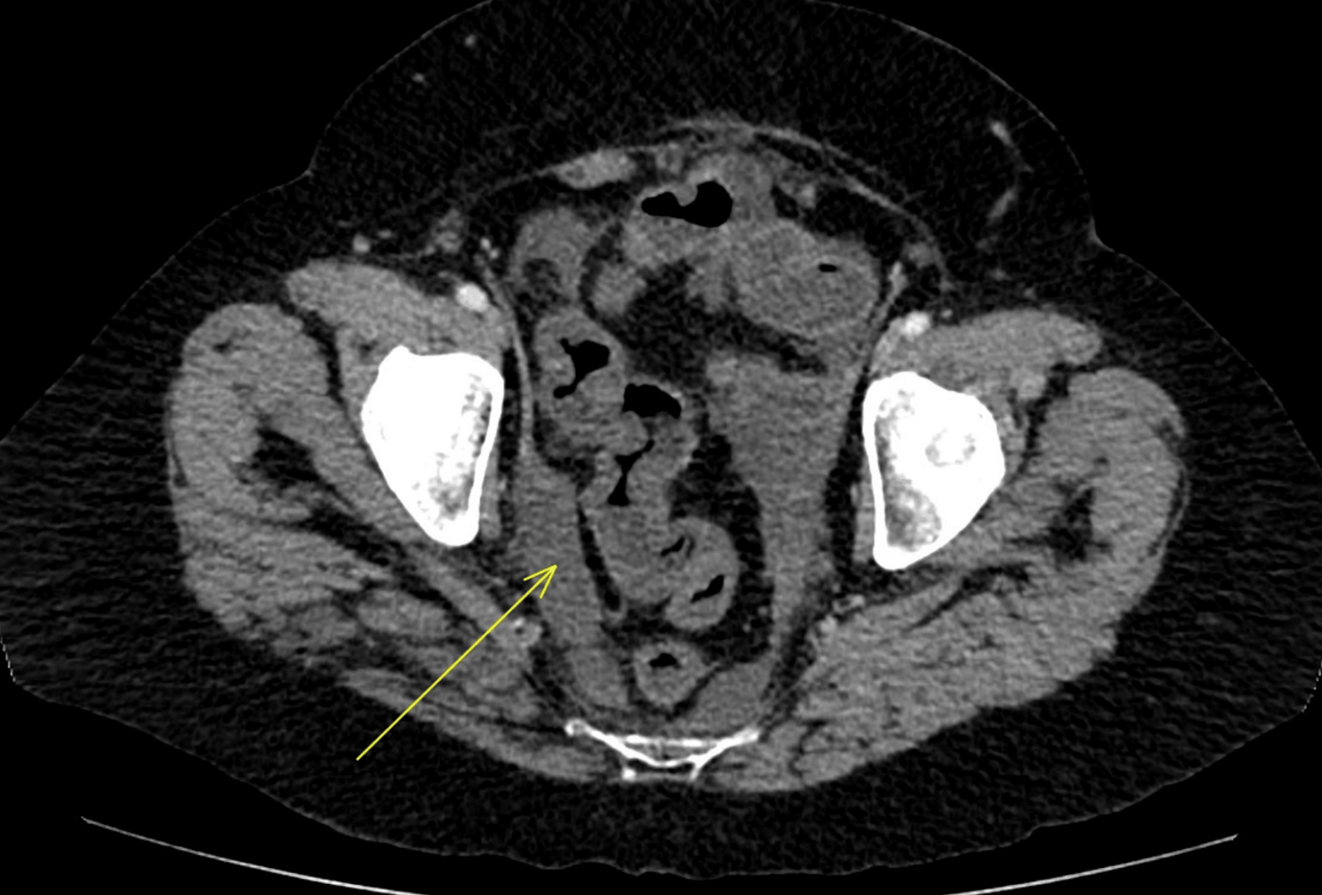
Axial view of abdominal CT showing increased bowel wall thickness, bowel mucosal enhancement, and ascites (arrow) CT: computed tomography
